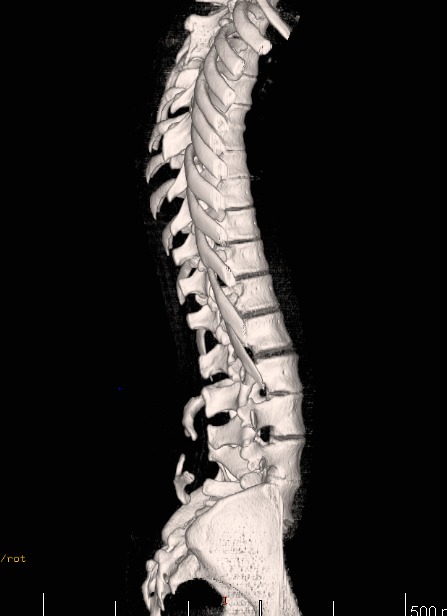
3D CT reconstruction in an 18-year-old patient: the lateral image reveals two vertebral arches arranged as crab forceps. L3 vertebral arch is positioned in a synchondrosis-shifting manner with the L2 spinous process. L4 vertebral arch is floating
